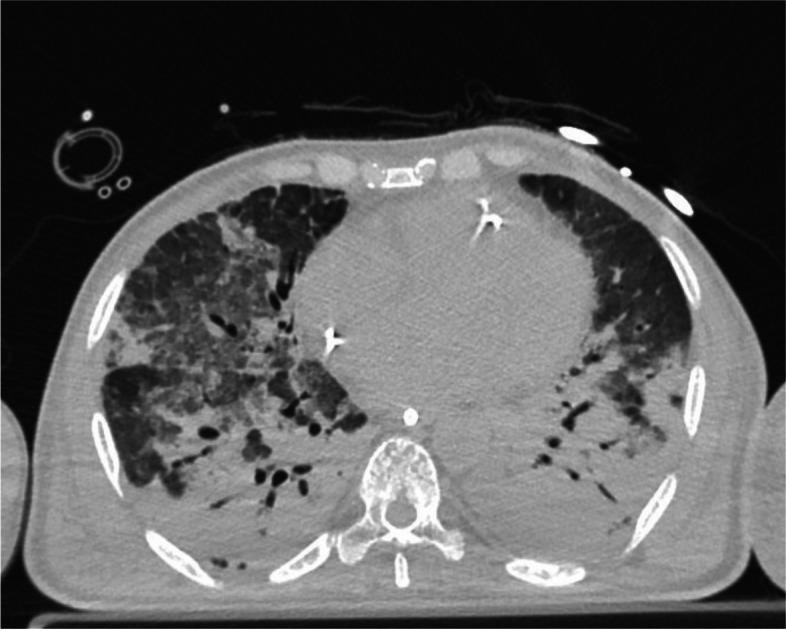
CT scan of an ARDS patient on V-V ECMO with a high shunt. In this figure, we report an exemplary lung CT image of a patient undergoing V-V ECMO for severe respiratory failure with a shunt of 69%. It is visible the massive consolidation of the basal bilateral lung regions and the ground-glass opacities of the middle and upper lung areas
